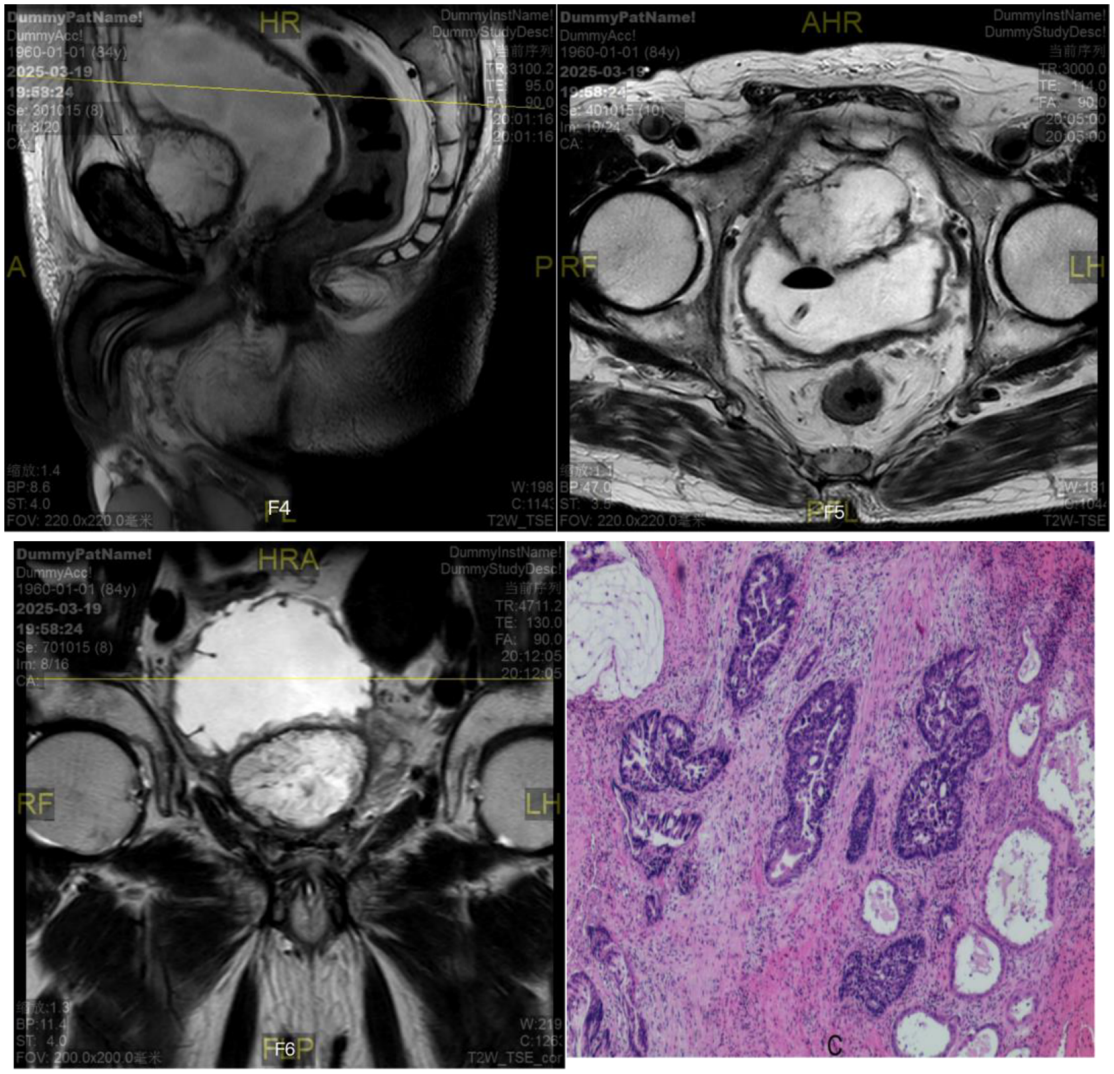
MRI of the prostate+the extraperitoneal mass was adenocarcinoma.
